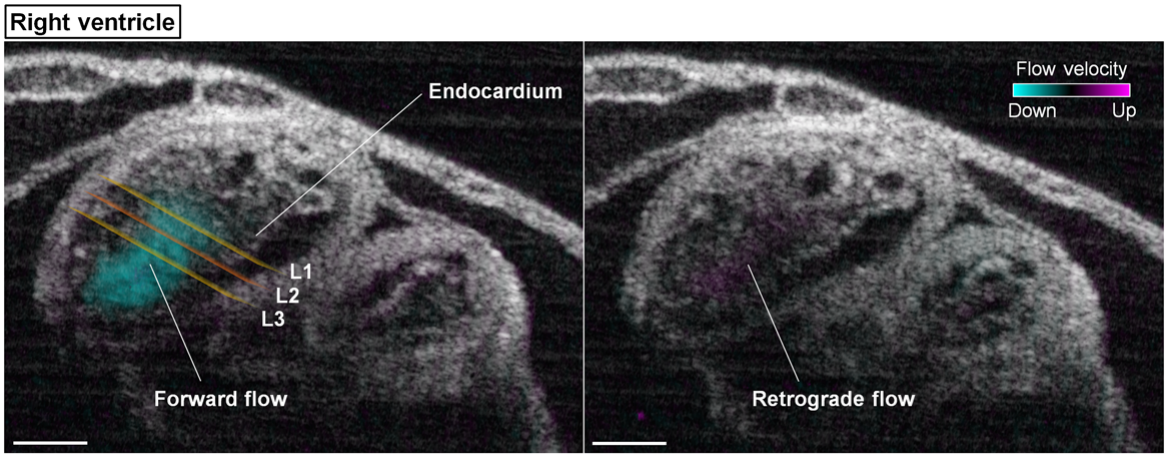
Structural and Doppler 4D-OCT imaging of the right ventricle of E9.25 embryonic heart (Video 2). The positions of the measurement planes L1, L2, and L3 are shown in the left panel. Scale bars are 100  μm (Video 2, mov, 8.59 MB [URL: https://doi.org/10.1117/1.JBO.25.8.086001.2]).
